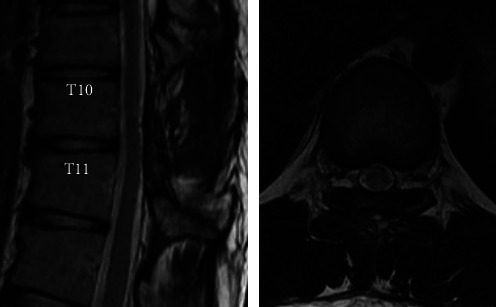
Magnetic resonance imaging one year after operation shows a completely resected and well-decompressed spinal cord.
